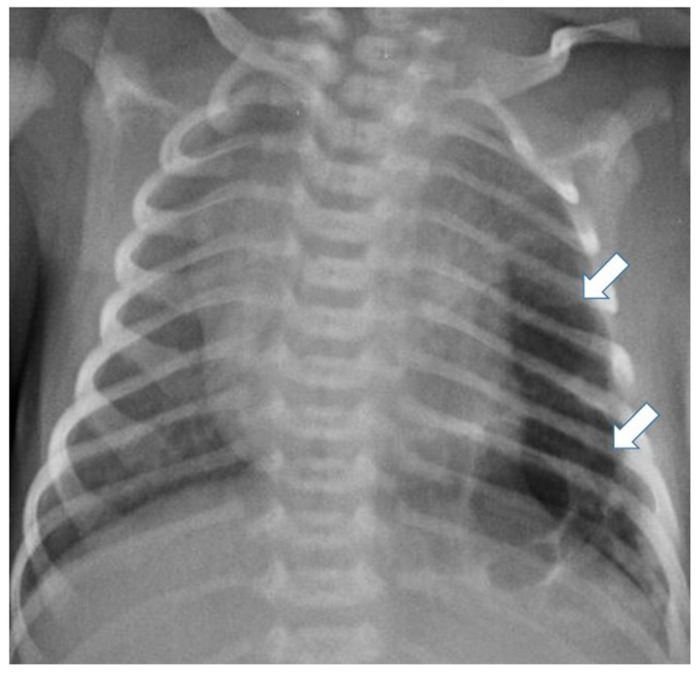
Chest radiograph of a newborn shows multiple large air-filled cysts in the left lower lobe (arrows), suggesting a type 1 CPAM.
